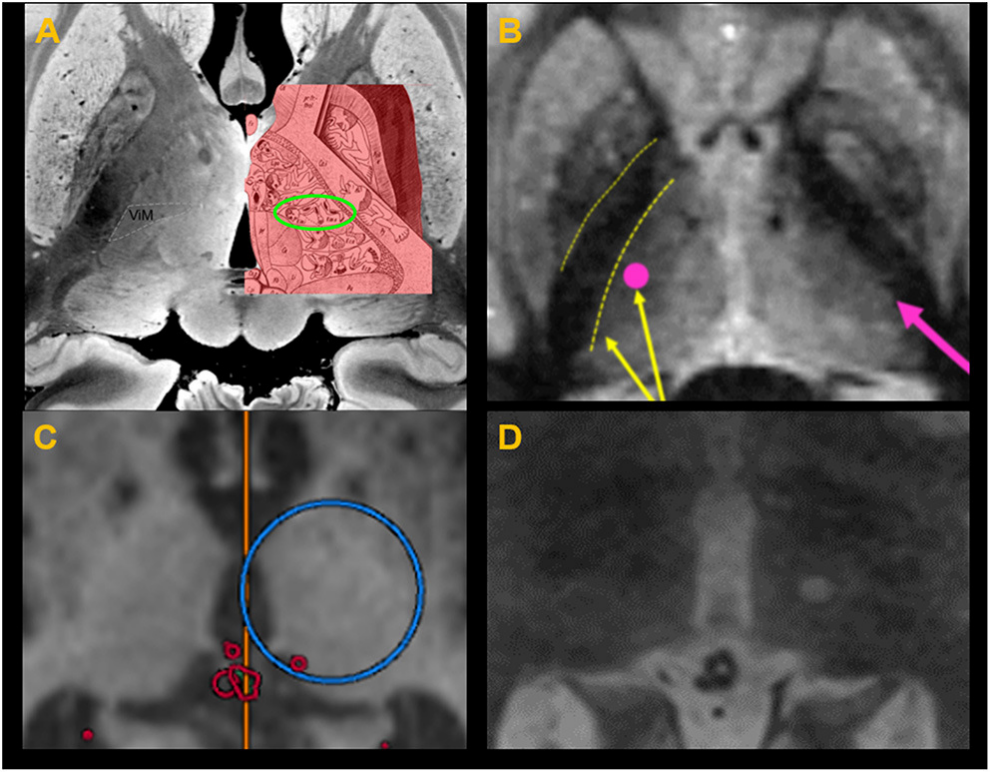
(A) Thalamic homunculus superimposed over the thalamus in post mortem ultra–high-resolution images obtained at 7T. The green circle indicates the location of the ventral intermediate nucleus (Vim). (B) Fast gray matter acquisition T1 inversion recovery scan shows sharp demarcation between the IC (internal capsule) and thalamus, allowing adjustment of the target selection to avoid lesion spread into the IC. (C) Target coordinate selection during treatment planning using the anterior commissure–posterior commissure as standard references for localizing the target. (D) A successful lesion is created at the Vim, confirmed with a T2-weighted image obtained immediately after sonication with the ablative temperature.
